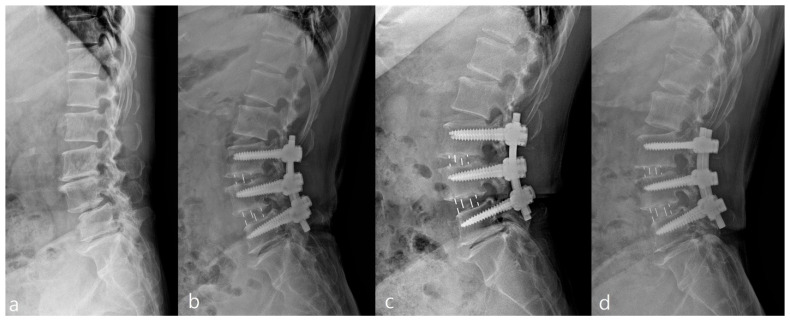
Upright lateral lumbar spine radiographs of a representative patient who underwent L3-5 OLIF. Images demonstrate early radiographic changes at different timepoints: (a) preoperative, (b) postoperative 1 month, (c) postoperative 3 months, and (d) postoperative 6 months.
